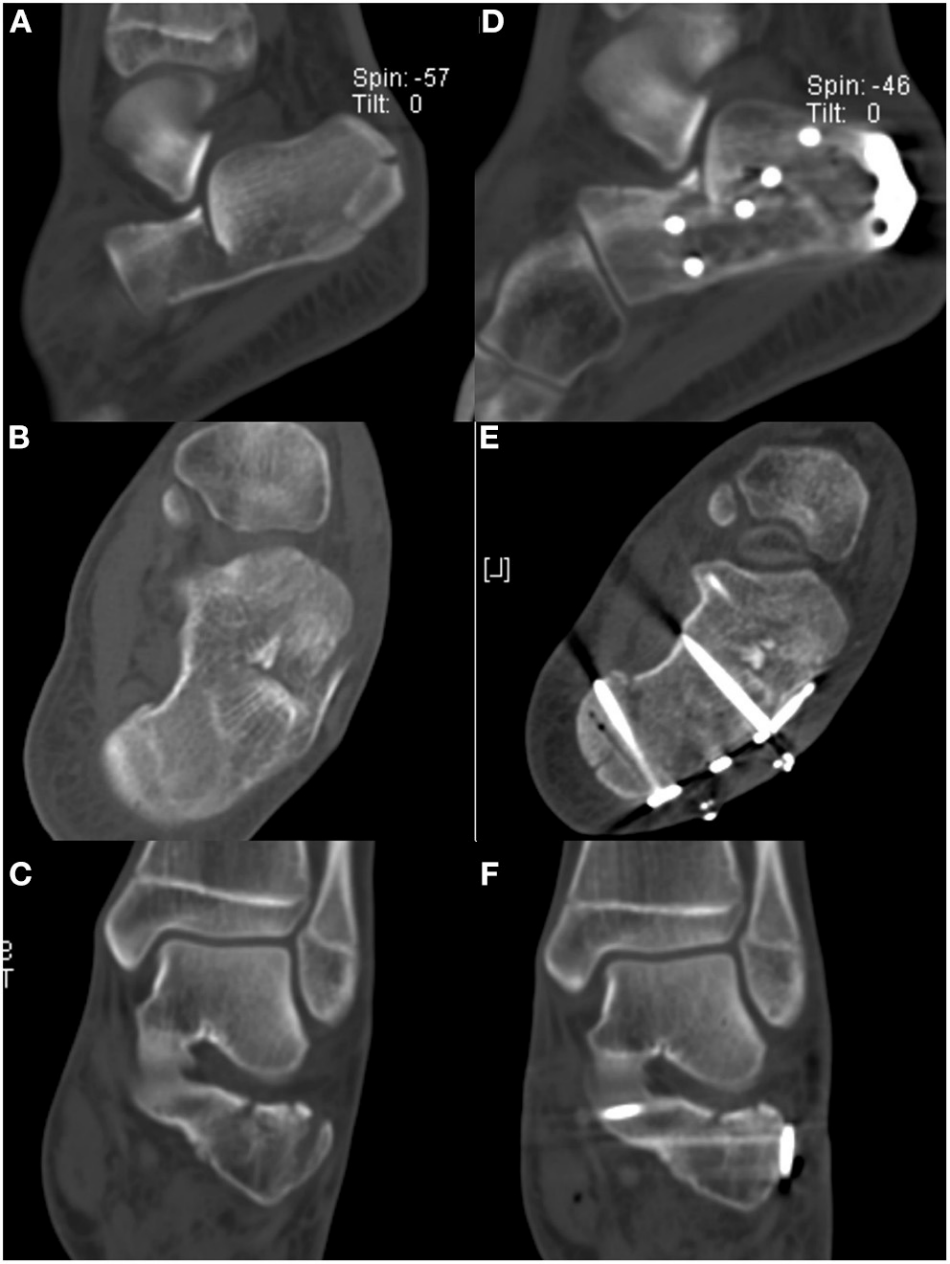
(A–C) The preoperative computed tomography (CT) scan of the foot from Figure 3 shows a Sanders type II fracture. (D–F) Postoperative CT scan shows the reduction of the articular surface and the restoration of the calcaneal width were satisfactory.
